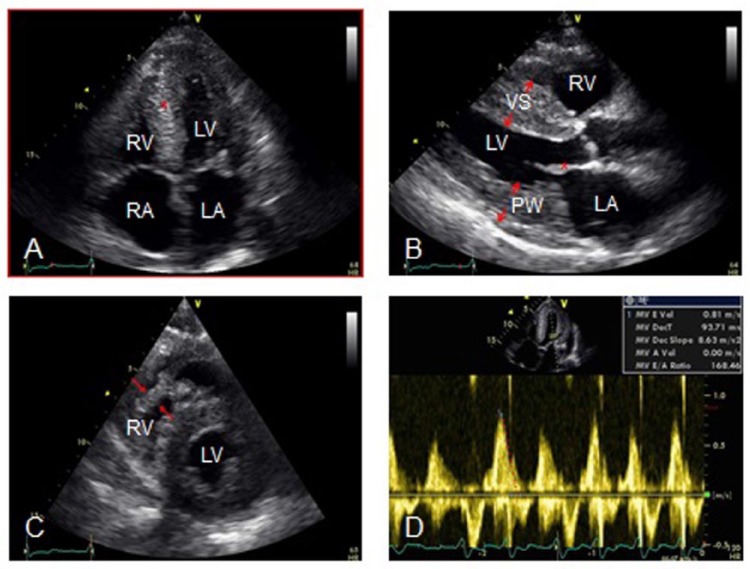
Two-dimensional echocardiogram. (A) Four-chamber view demonstrating bi-atrial enlargement (RA and LA) with speckled granular appearance of the myocardium (asterisk). (B) Parasternal long axis view demonstrating thickened ventricular septum (VS), posterior wall (PW), and thickened mitral valve leaflets (asterisk). (C) Short axis view demonstrating right ventricular hypertrophy (arrows). (D) Mitral valve inflow velocities demonstrating rapid deceleration time consistent with severe restrictive physiology. Abbreviations: RA, right atrium; LA, left atrium; RV, right ventricle; LV, left ventricle; VS, ventricular septum; PW, posterior wall.
